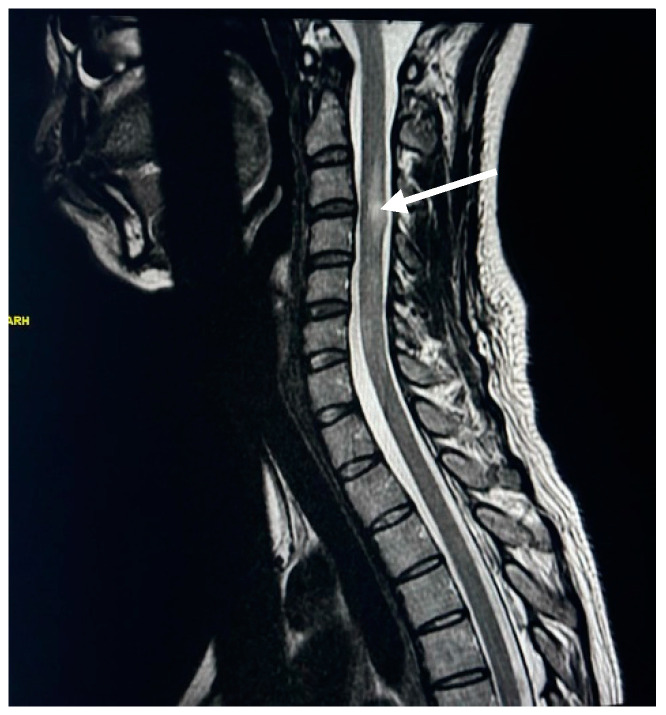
T2W MR image of cervical spine plaque. The white arrow demonstrates a high signal intensity representing cervical plaque.
